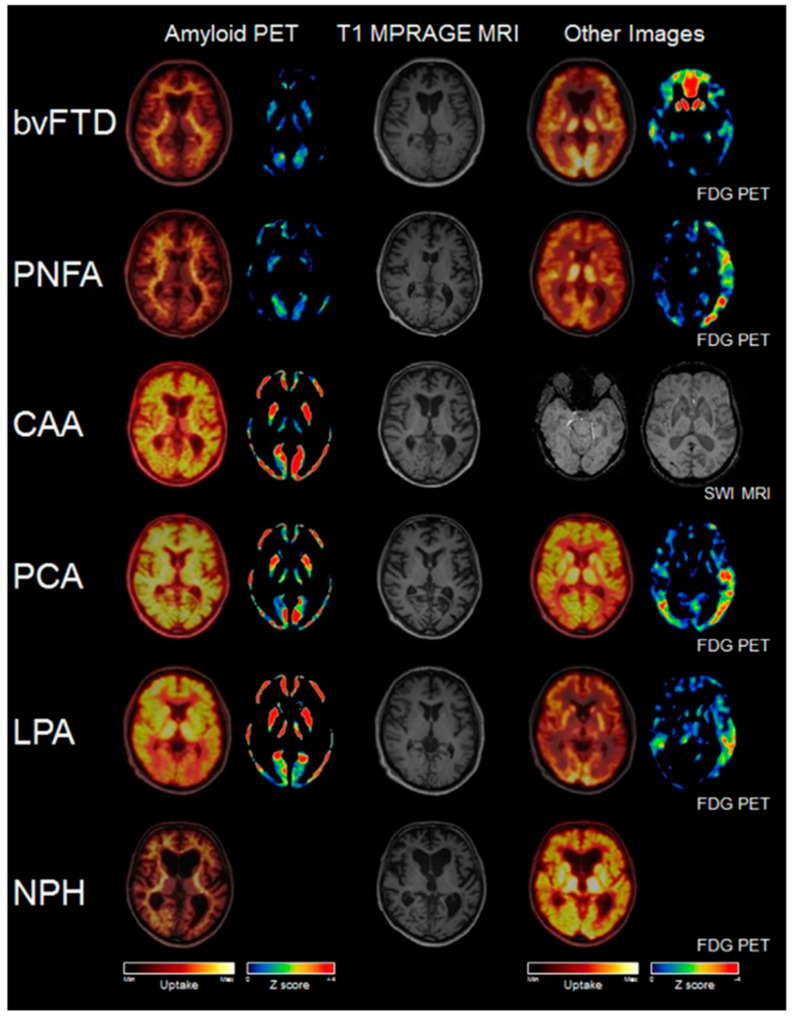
Typical amyloid PET/MRI in rare dementia diseases are shown in the image. The image consists of two columns on the left side, displaying the overlay of 18F-florbetaben amyloid PET/MR. The middle column shows the corresponding anatomical T1-MPRAGE MR slice. The right side of the image displays the corresponding slices of other imaging modalities. The abbreviations used in the image include bvFTD for behavioral-variant frontotemporal dementia, CAA for cerebral amyloid angiopathy, LPA for logopenic aphasia, NPH for normal pressure hydrocephalus, PCA for posterior cortical atrophy, PNFA for progressive nonfluent aphasia, and SWI for susceptibility-weighted MRI [60].
